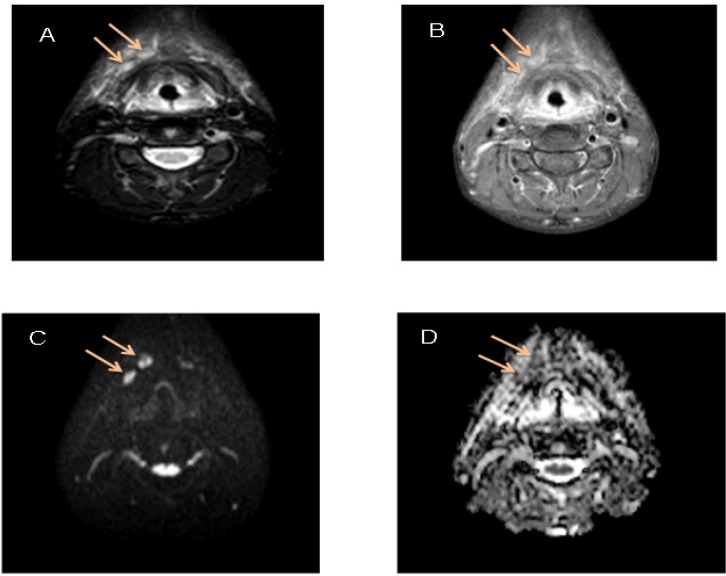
A 17 Year-Old Male with Thyroid Cancer. A, STIR shows two enlarged right neck LNs; B, T1W post contrast shows enhanced LNs; C, DWI shows high signal intensity; D, ADC displays restricted diffusion of LNs on ADC (0.865 × 10^-3^ mm^2^/s)
